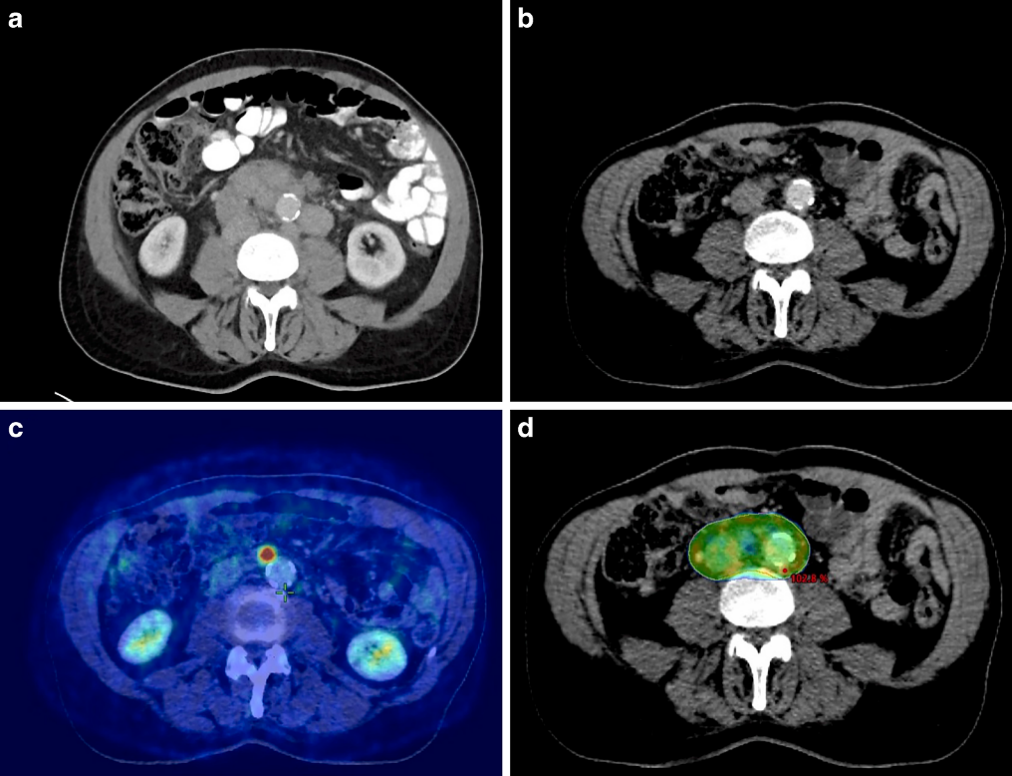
Consolidative radiotherapy of positron emission tomography (PET-)positive residues. a Initial (prechemotherapy) CT scan revealing an abdominal bulk of DLBCL. b Postchemotherapy CT scan of the abdomen showing an excellent response with only minimal residues. c F^18^-FDG-PET-CT indicates metabolically active tumor (Deauville 4) despite the good morphological response. d Colorwash representation of the 95% isodose covering the planning target volume (*thin orange line*). Radiation treatment was delivered via a volumetric modulated arc therapy photon plan
